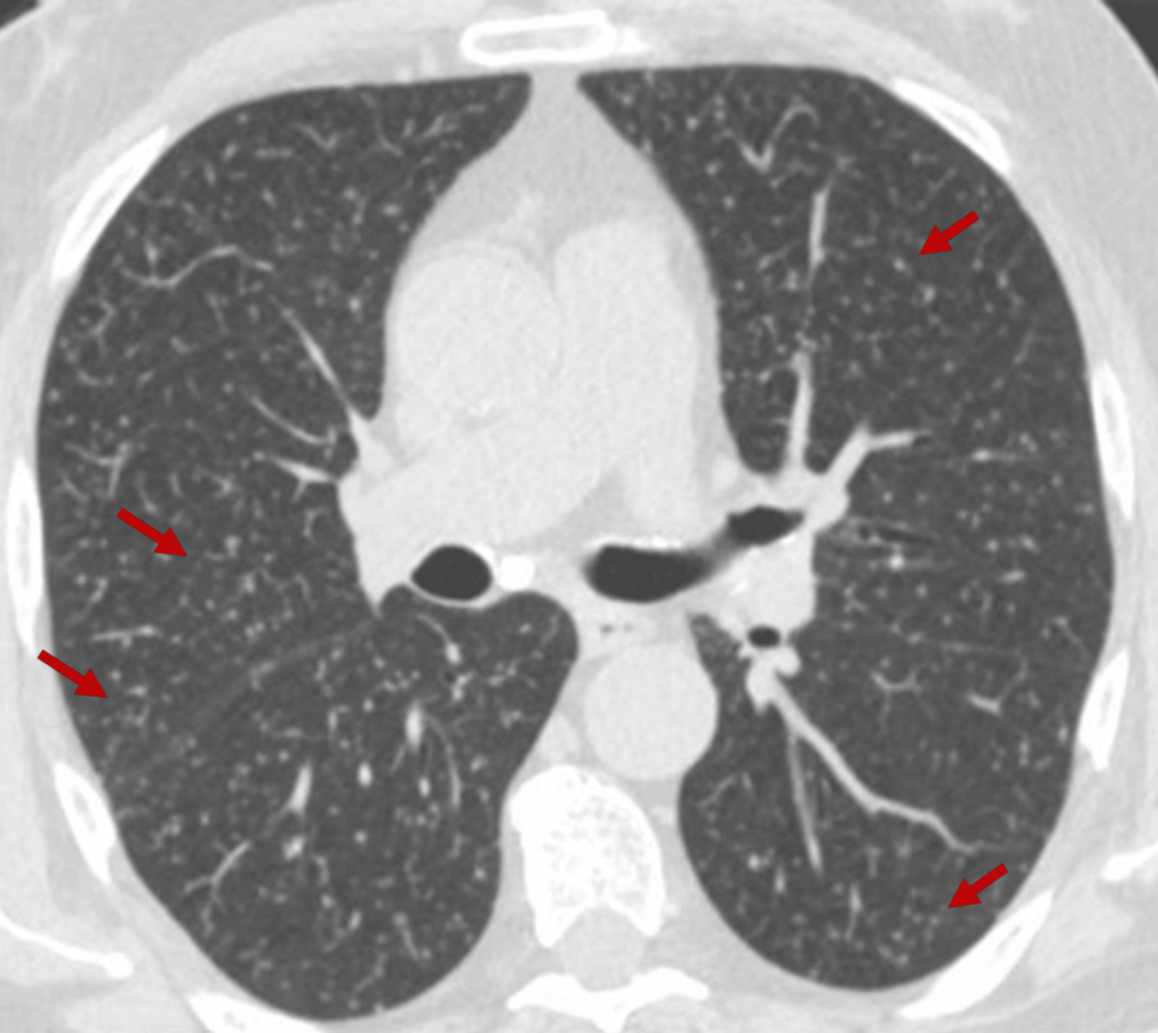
Chest CT showing diffuse bilateral micronodular infiltration in a miliary pattern (arrows).
